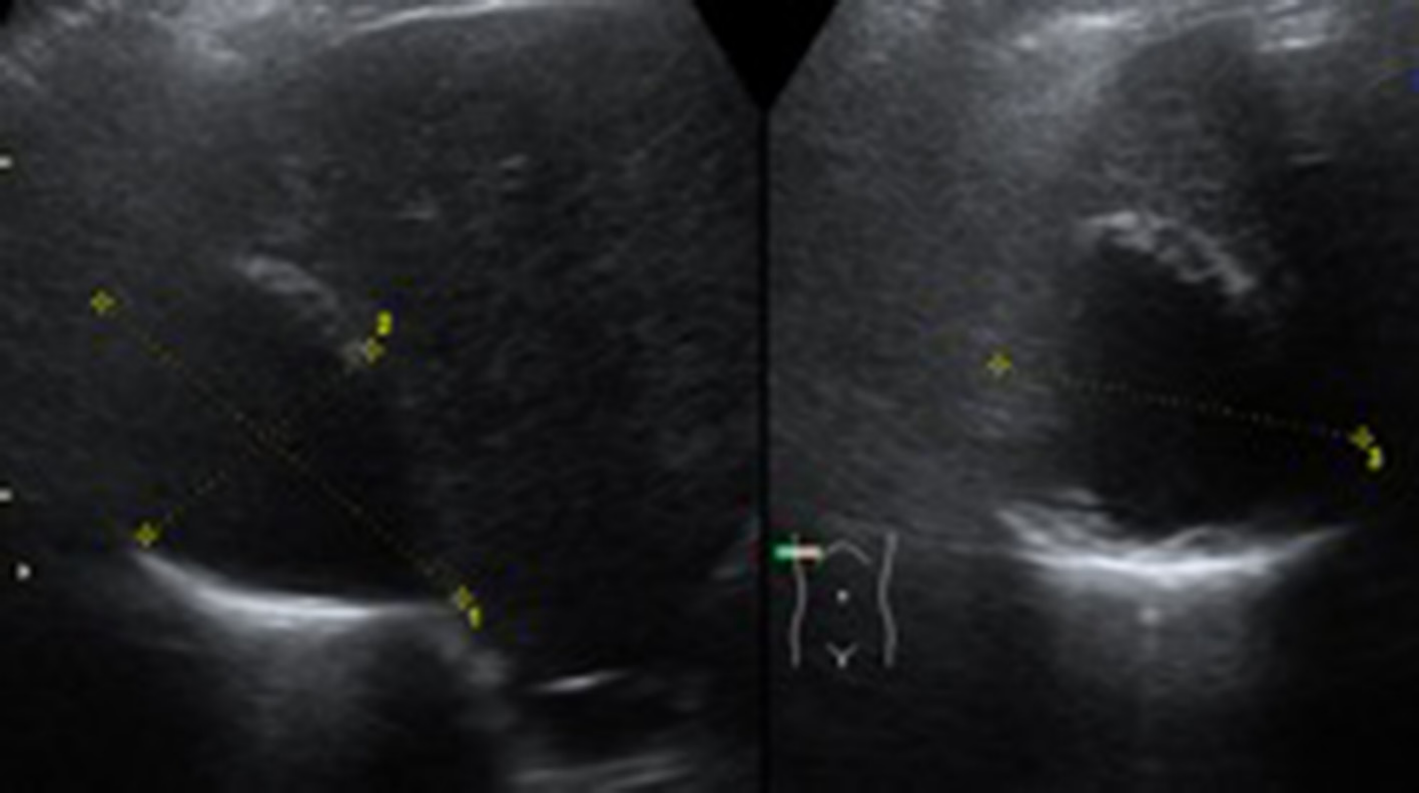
Ultrasound shows right upper quadrant masses with hypoechoic and hyperechoic components.
